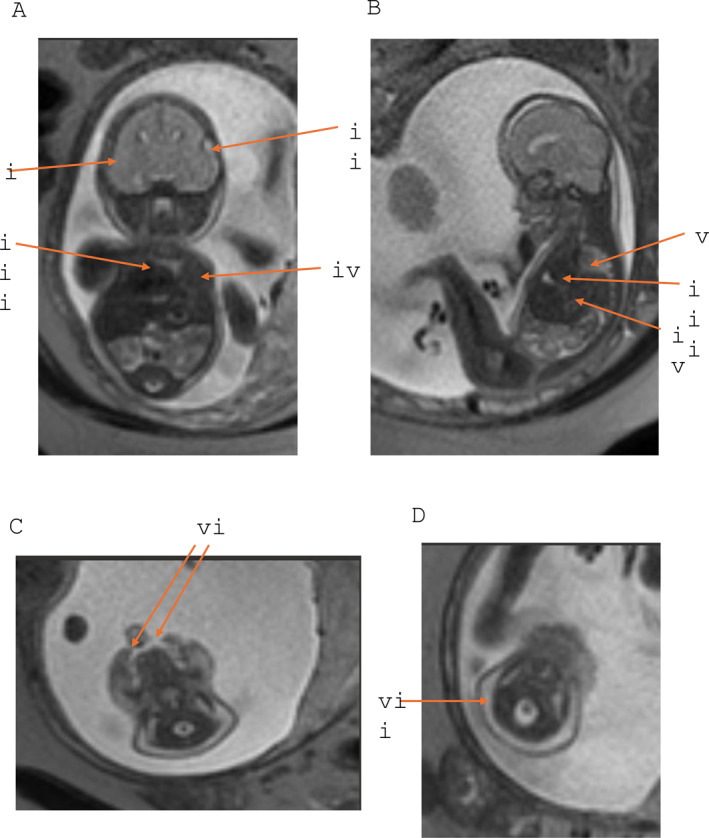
A–D: Fetal MRI images. Figure 1A is a coronal plane view of the head and brain showing turricephalic head (i) and significantly reduced extra axial CSF space (ii). Figure 1B is a sagittal plane view. The two views demonstrate a right shifted heart (iii) and liver herniation into the chest cavity (iv). Hypoplastic lung (v) due to the herniating liver is shown in Figure 1B. Figure 1C is an axial view of the fetal head demonstrating a cleft palate (vi). Figure 1D is an axial view of the fetal neck and inferior head displaying the cystic hygroma (vii).
